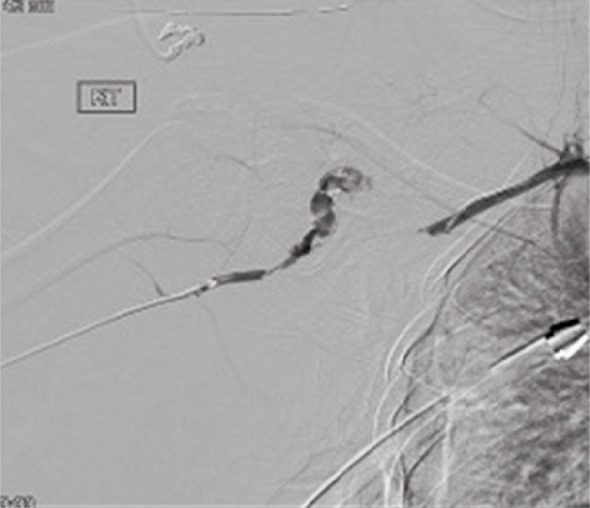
CTA showing subclavian artery long segment (60mm) avulsion with contrast extravasation. Minimal collaterals noted with reconstitution of the distal axillary artery.
